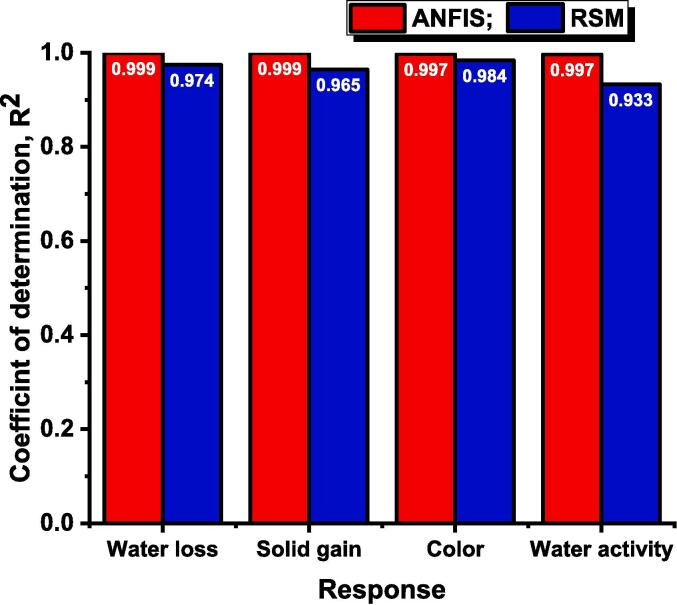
Coefficient of determination of RSM and ANFIS model designed for ultrasound assisted osmotic dehydration of cape gooseberry.
